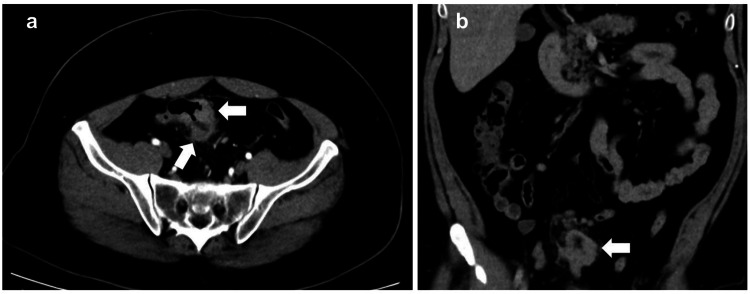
The contrast-enhanced computed tomography (CE-CT) images The arrows of sub-images a and b reveal the sigmoid colon wall thickening, and pathologic biopsy results showed adenocarcinoma. Images a and b shown in cross and coronal sections, respectively.
